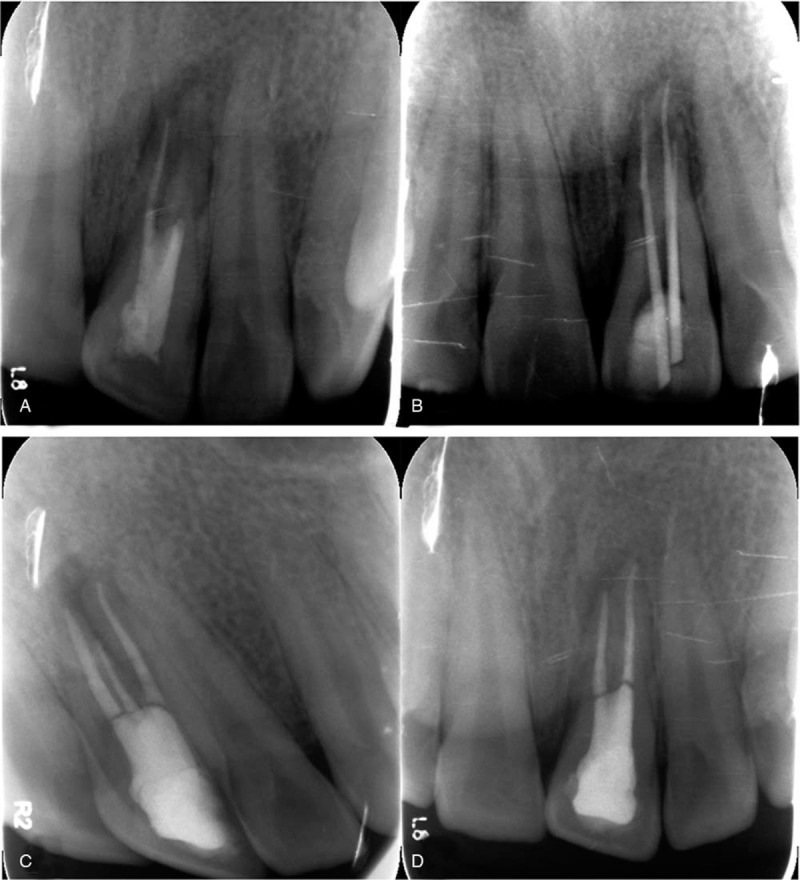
(A) The preoperative, (B) intraoperative, and (C and D) postoperative radiograph of #21 tooth.
